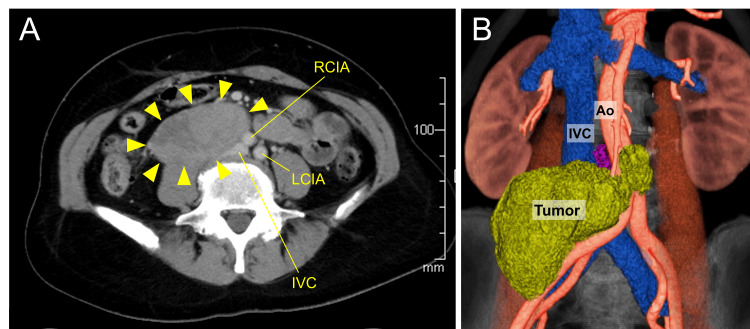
Contract-enhanced computed tomography (CT) showing retroperitoneal tumor (A) Axial view of CT showed a 5 × 4 × 7.5 cm iso-density mass in the right retroperitoneum (arrowheads). (B) On 3D reconstruction images of CT, the Ao and IVC are compressed by the tumor. Ao: aorta, IVC: inferior vena cava, LCIA: left common iliac artery, RCIA: right common iliac artery
